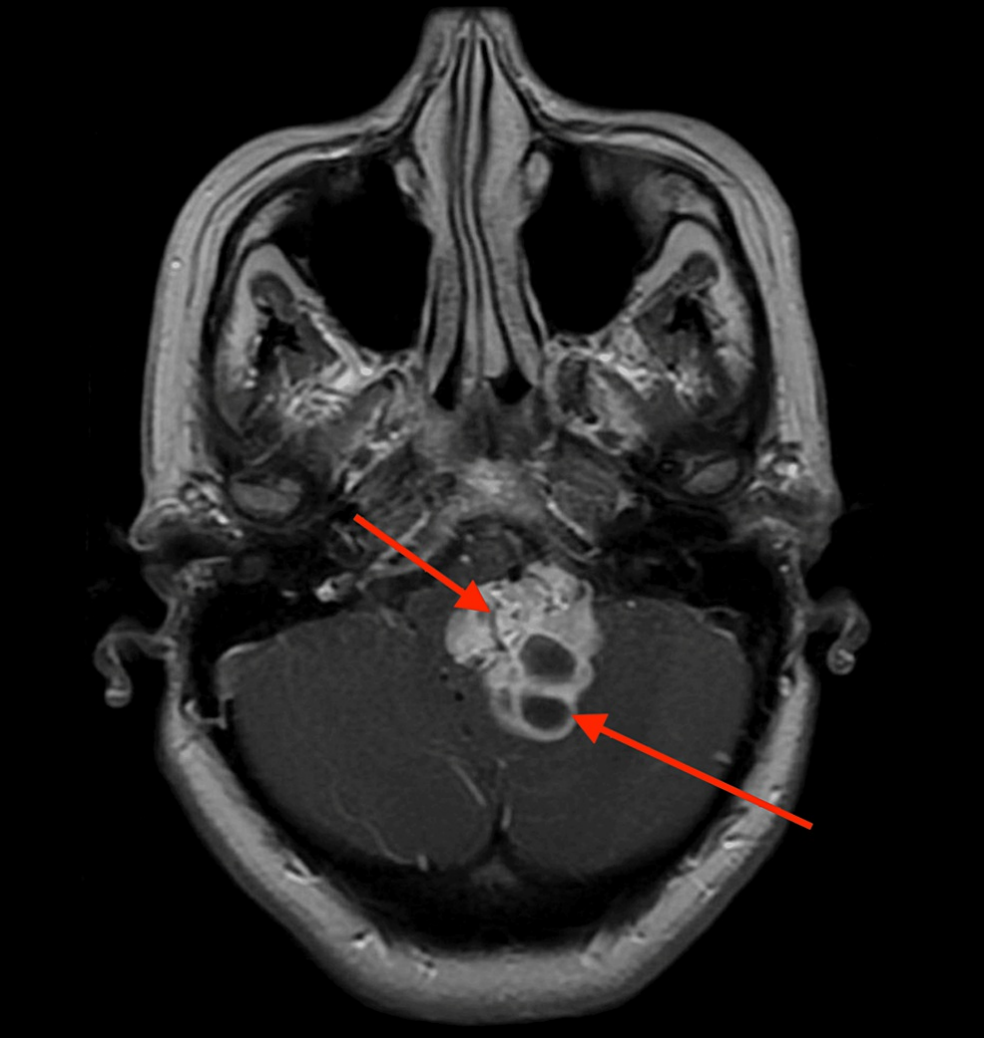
Post-contrast T1-weighted MRI reveals an irregular, mixed solid and cystic avidly enhancing mass centered in the foramen of Luschka that measured approximately 3.5 x 3.0 x 4.2 cm​
